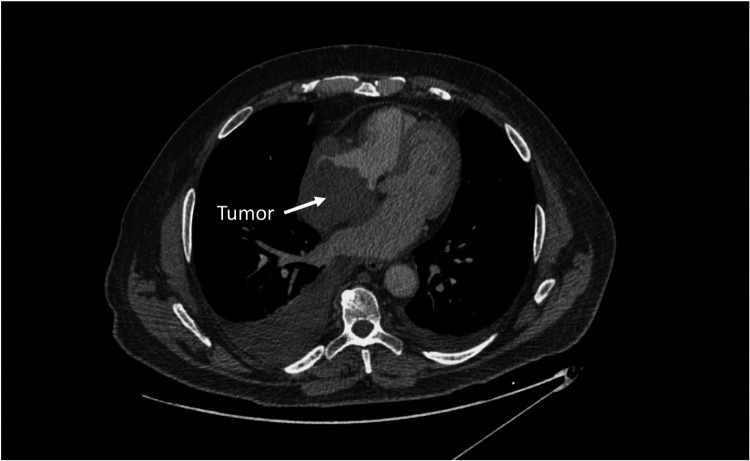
Chest CT scan demonstrating a large right atrial mass.
